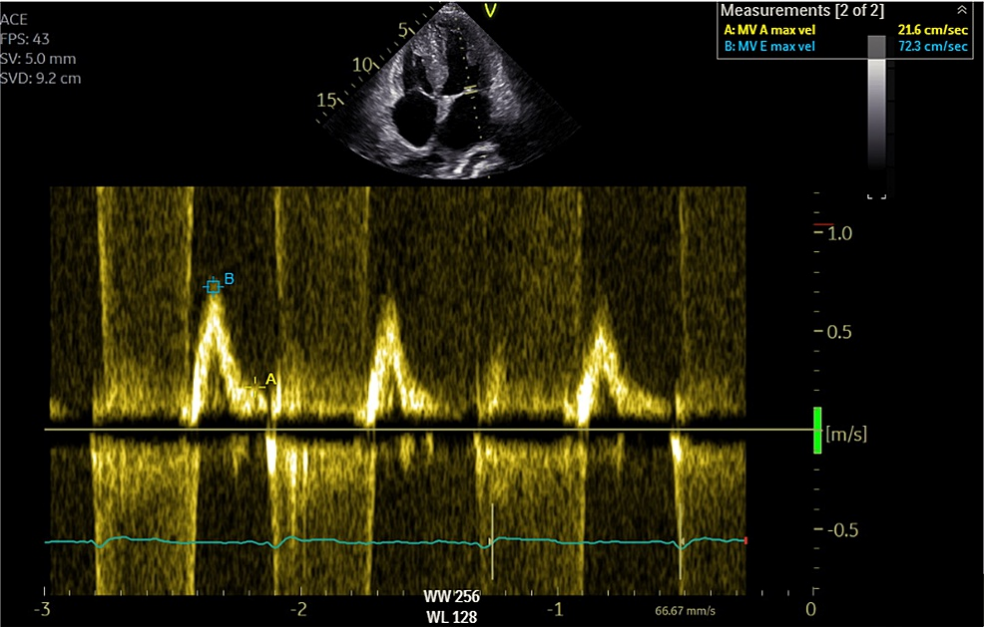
Echocardiography showing grade III diastolic dysfunction with an E/A ratio superior to 2. B = E wave; A = A wave
